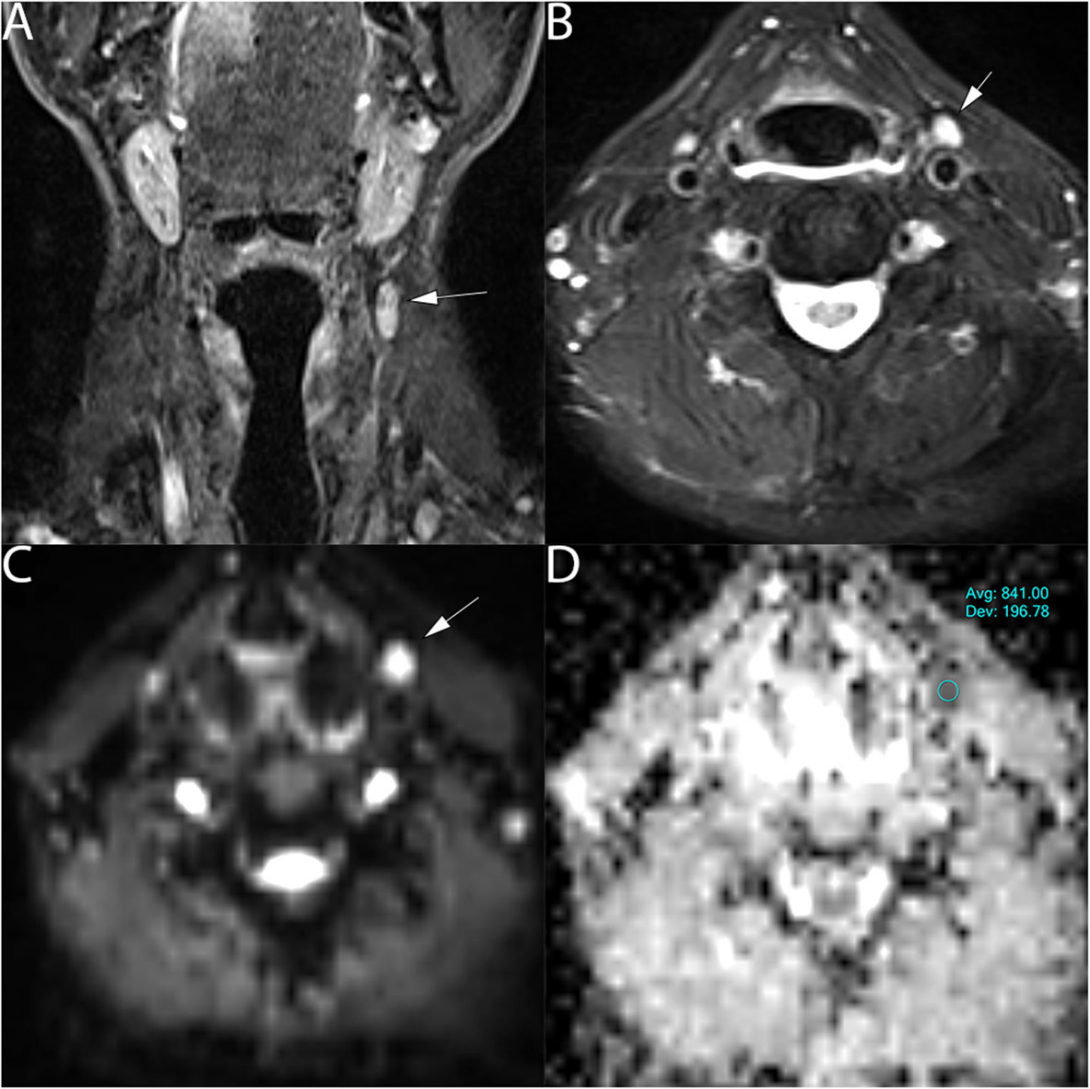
65 years old male with para-pharyngeal sub-centimeters lymph node positive malignancy from laryngeal SCC on the left side of the neck. a T2 coronal show the lymph node, (arrow). (B) T2 axial show the axial extension of the lymph node (6x8mm), (arrow) (c) DWI show hyperintense area at b value 800mm^2^/s (arrow) and (d) ADC map on the targeted lymph node show hypointense signal, an ADC value of (0.841 ± 0.19*10^− 3^ mm^2^/s)
